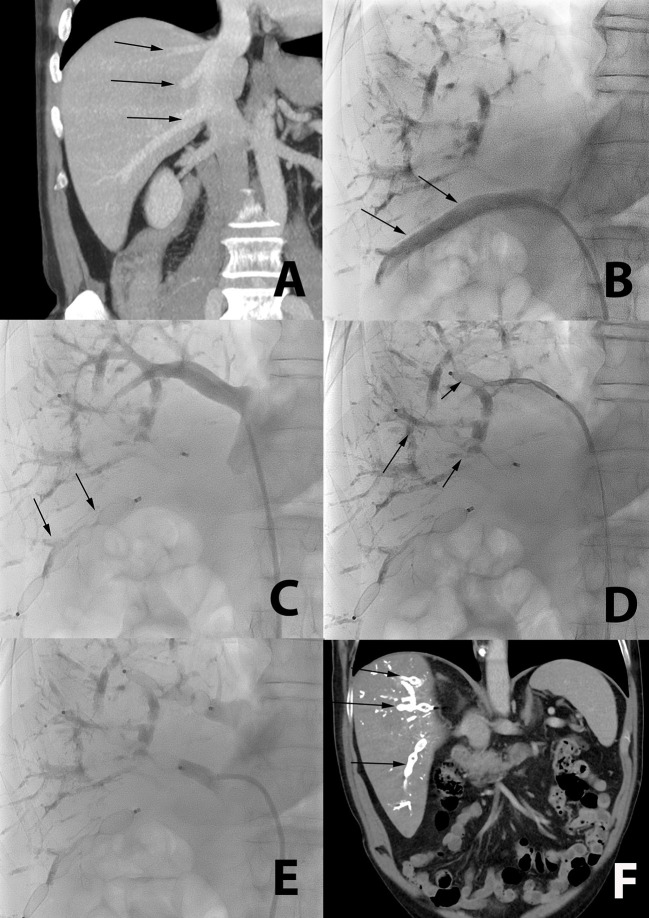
Occlusion of multiple accessory veins using a single femoral access in a 72-year-old patient with liver metastasis from colorectal cancer. (A) CT coronal reformation of a patient with large segmental inferior right hepatic vein (lower arrow), and two smaller segmental veins (middle and upper arrow), all draining directly in the inferior vena cava. (B) Catheterization (arrow) of the large segmental inferior hepatic vein using a femoral access. (C) Plugs are deployed in this large segmental inferior vein (arrow). (D,E) The two other segmental veins are subsequently catheterized and occluded with plugs (arrow). (F) Post-mLVD CT coronal reformation showing occlusion of all segmental veins with the plugs (arrows).
